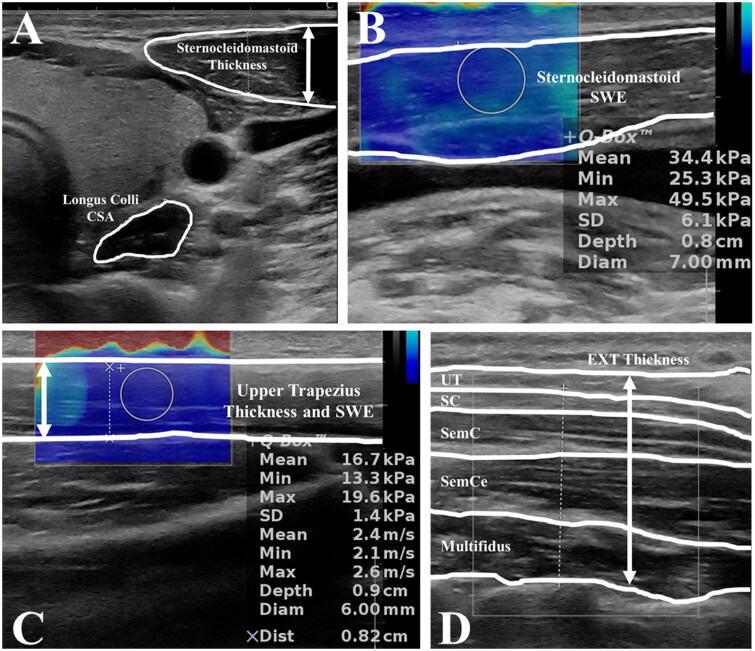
Ultrasound images: A. the sternocleidomastoid thickness and the longus colli cross-sectional area (CSA); B. the sternocleidomastoid shear wave elastography (SWE); C. the upper trapezius thickness and shear wave elastography; and D. the cervical extensors (EXT) thickness.
